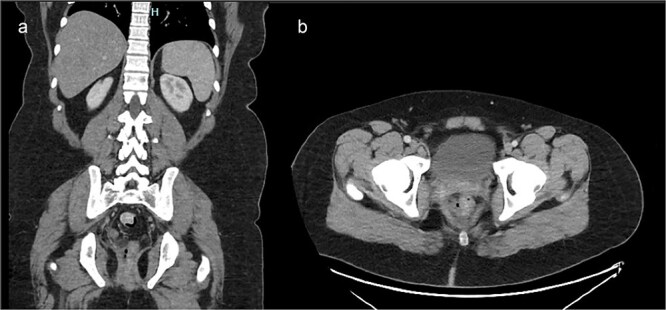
Contrast-enhanced CT of the pelvis. (a) Axial image demonstrating inflammatory stranding and perirectal fluid with foci of extraluminal air adjacent to the posterior rectum. (b) Coronal image showing disruption of the posterior rectal wall with associated soft tissue changes extending into the presacral space.
